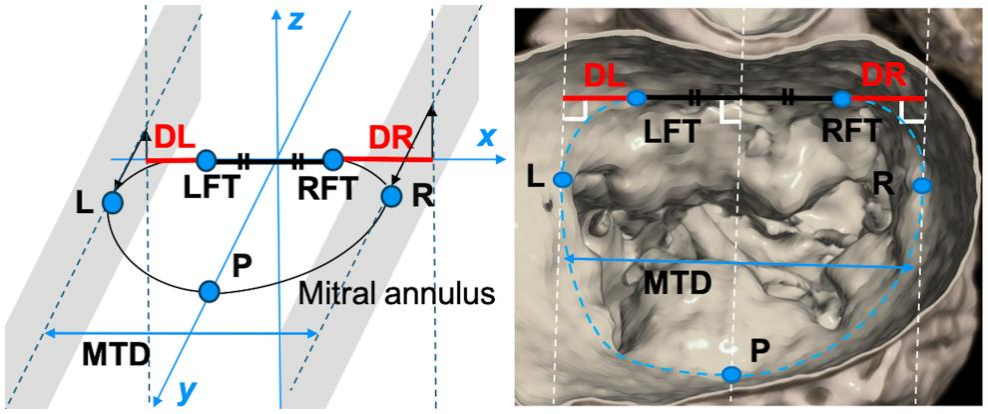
Mitral annulus analysed using three-dimensional computed tomographic reconstructions. The definition of the three-dimensional Cartesian coordinates in this study is as follows: The origin is the midpoint of the left fibrous trigone and the right fibrous trigone. The *XY* plane is the plane passing through the left and right fibrous trigones and point P on the mitral annulus. Point P is the intersection of the plane bisecting the left and right fibrous trigones perpendicularly and the mitral annulus. In the preceding coordinate system, the distance from the left fibrous trigone to the plane parallel to the *YZ* plane passing through the leftmost mitral annulus and the distance from the right fibrous trigone to the plane parallel to the *YZ* plane passing through the rightmost mitral annulus were measured. The blue dotted line in the right panel indicates the mitral annulus. DL: distance from the left fibrous trigone to the plane parallel to the YZ plane passing through the leftmost mitral annulus that had the smallest X-value among all points of the mitral annulus; DR: distance from the right fibrous trigone to the plane parallel to the YZ plane passing through the rightmost mitral annulus that had the largest X-value among all points of the mitral annulus; L: leftmost mitral annulus; LFT: left fibrous trigone; MTD: mitral transverse diameter; P: point on the mitral annulus; R: rightmost mitral annulus; RFT: right fibrous trigone.
